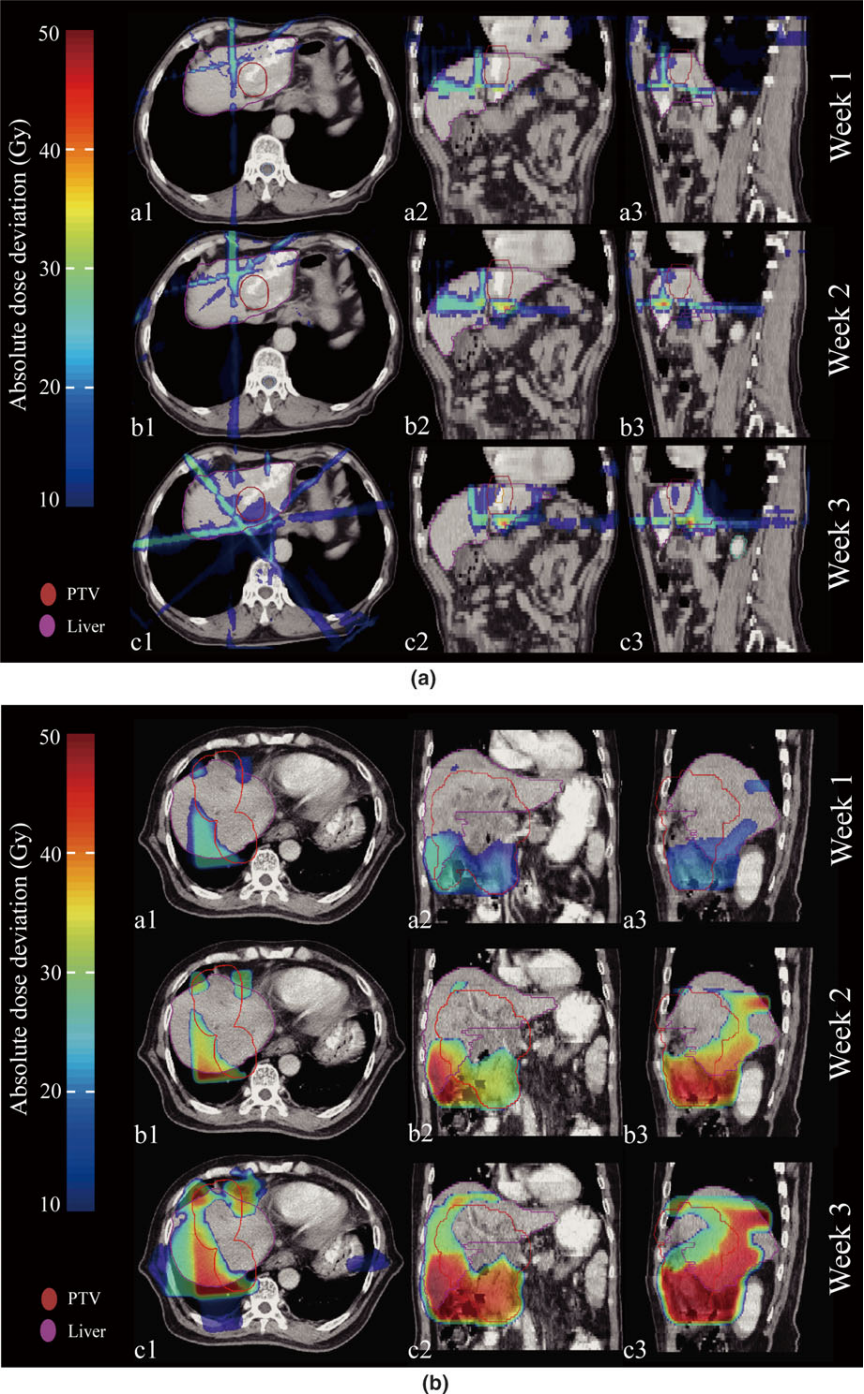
Example of absolute dose deviation on pCT for patients without (a) and with (b) RILD. The dose deviation between pCT and mCBCT was shown in three different periods (top for week1, middle for week2 and bottom for week3), and a fractional reconstructed dose deviation from the planned dose (D_mcbct_–D_plan_) are shown in the transverse (a1, b1, and c1), coronal (a2, b2, and c2), and sagittal (a3, b3, and c3) views, respectively. Each mCBCT was deformed to the pCT for dose subtraction.
